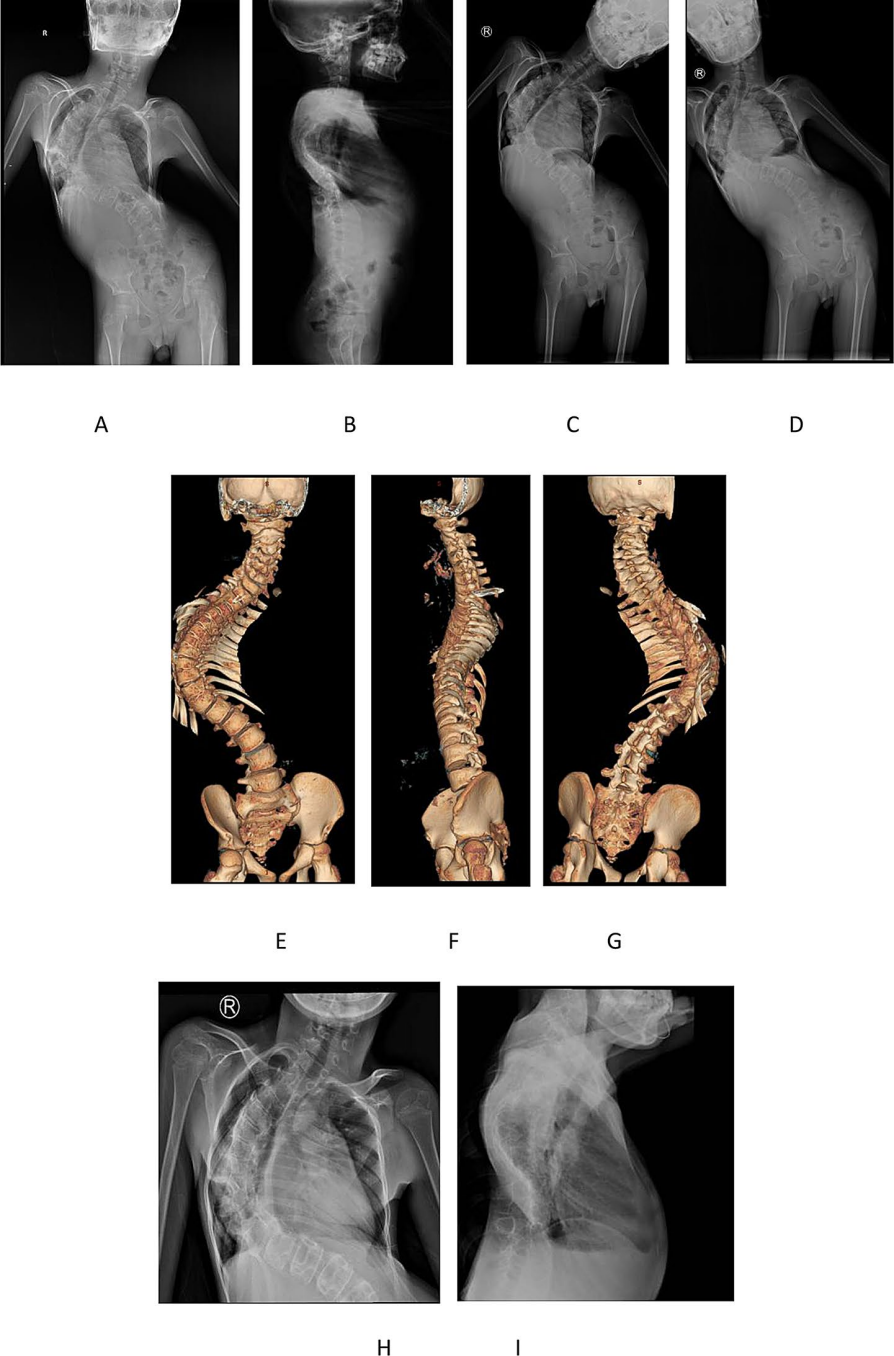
X-ray, CT images of the patient (A, B) The anteroposterior and lateral view of spinal X-ray. (C, D) The left and right bending view of spinal X-ray. (E, F, G) The three-dimensional CT images of spine. (H, I) The anteroposterior and lateral view of chest X-ray
